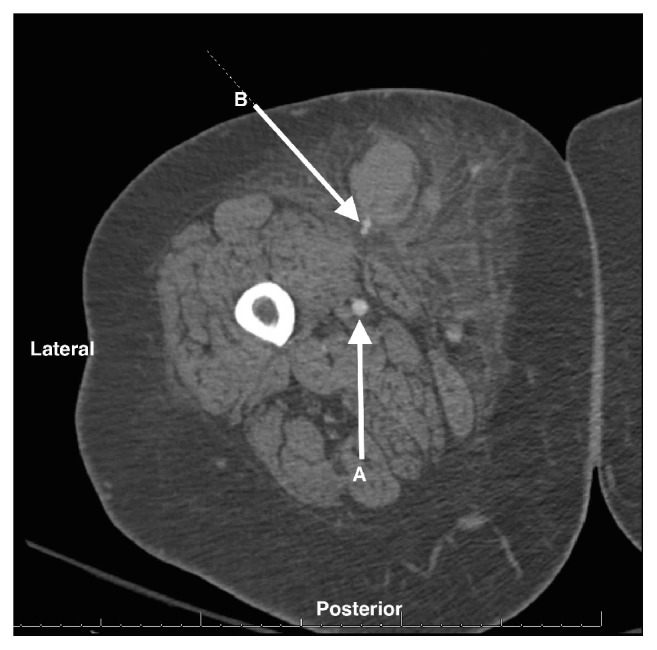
CT angiogram of the patient's right thigh showing the superficial femoral artery (A) and active extravasation from a small branch of the SFA (B).
